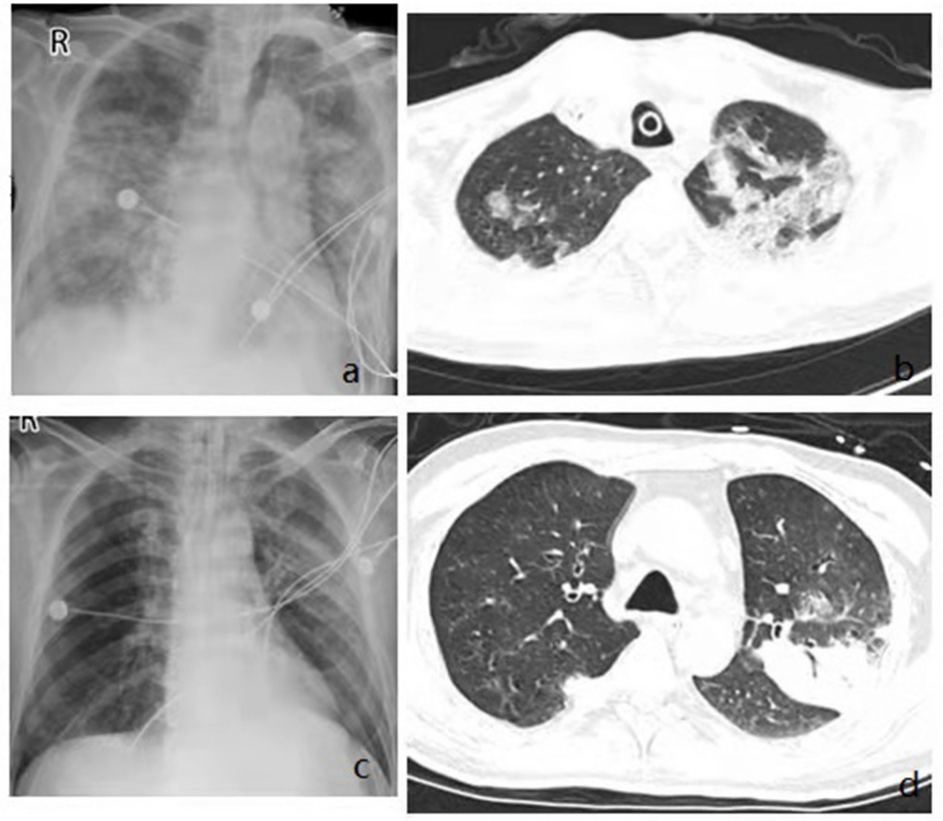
Imaging findings showing diffuse multifocal or diffuse opacity. Chest x rays from admission showing bilateral diffuse infiltrates (a,c), Chest CT from admission showing multifocal consolidation and groundglass opacity in both lungs (b,d).
